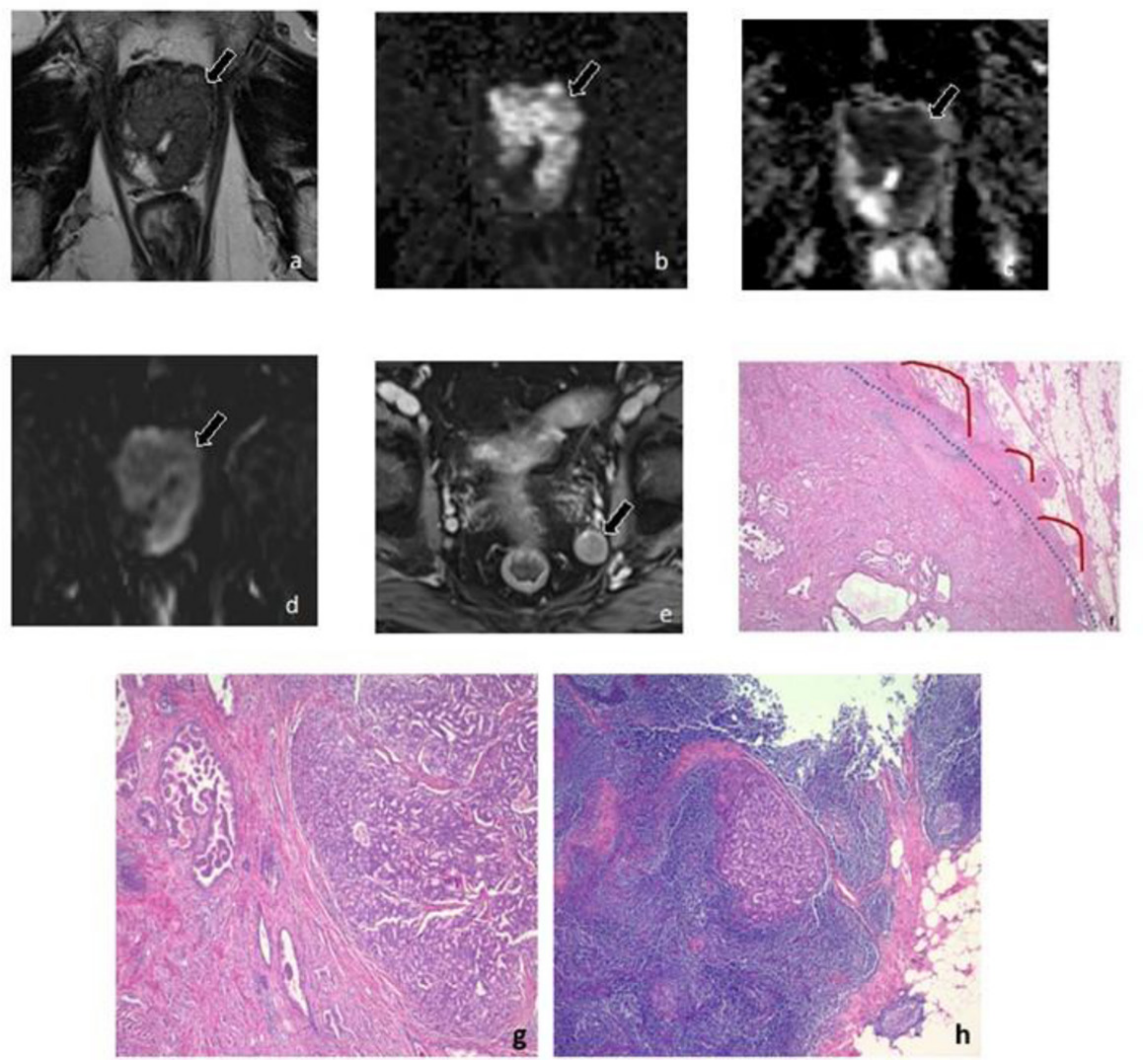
Patient with MRI-visible PLNM. A 70-year-old man had a prostate-specific antigen level of 69.4 ng/mL and ISUP-GG 3 prostate cancer on biopsy. (A) Axial *T*
